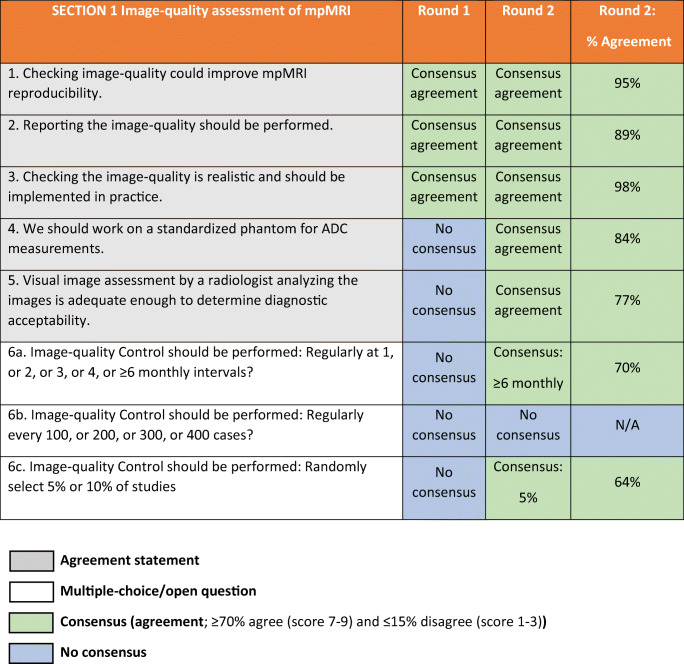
ESUR/ESUI consensus outcomes for section 1: Image quality assessment of mpMRI. *ADC* apparent diffusion coefficient, *ESUI* EAU Section of Urologic Imaging, *ESUR* European Society of Urogenital Radiology, *mpMRI* multi-parametric MRI, *N/A* not applicable
